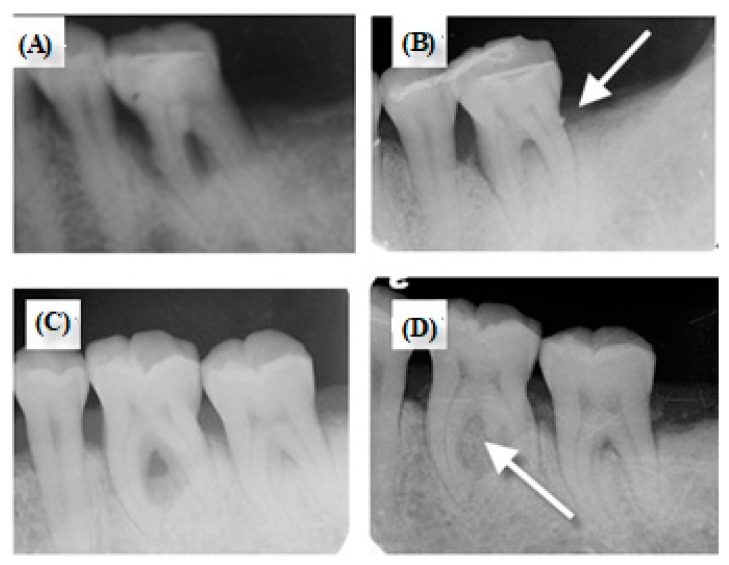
The patient A is with (A) pre-surgical radiograph (Tooth No. 36), (B) post-surgical radiograph with Epi-Guide^®^ membrane after 6 months. The patent B is with (C) pre-surgical radiograph (Tooth No. 37), (D) post-surgical radiograph with ES PLA95/β-TCP membrane after 6 months. Arrow (white) indicates the bone regeneration.
